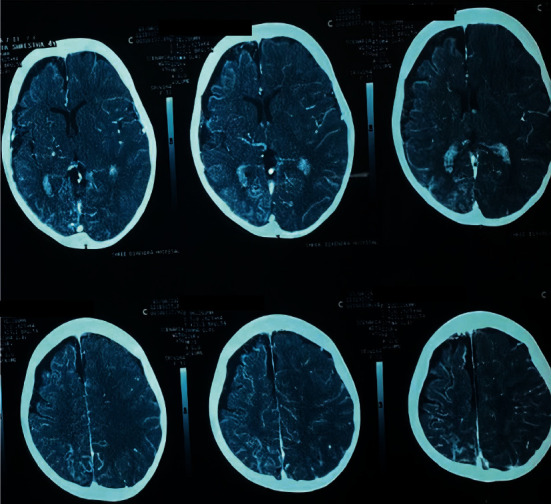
CT head (with angiography) showing volume loss of right brain parenchyma and linear calcifications.
